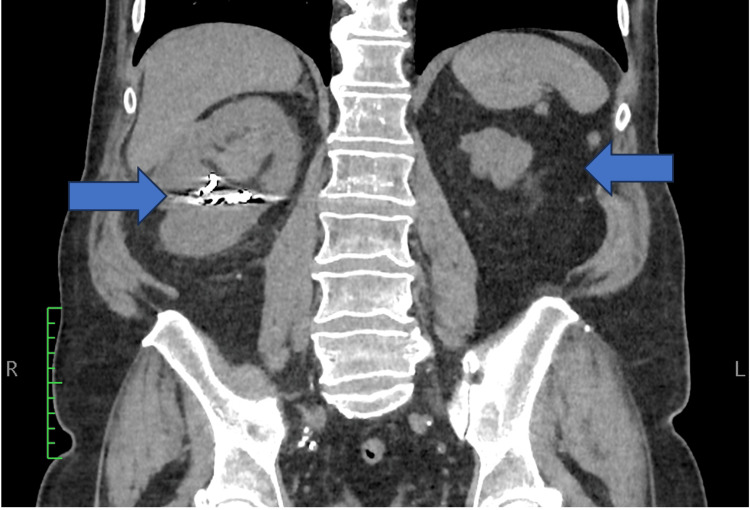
The preoperative CT scan of the patient showing left renal dysgenesis and a right kidney which has undergone previous partial nephrectomy (with clips on it).
